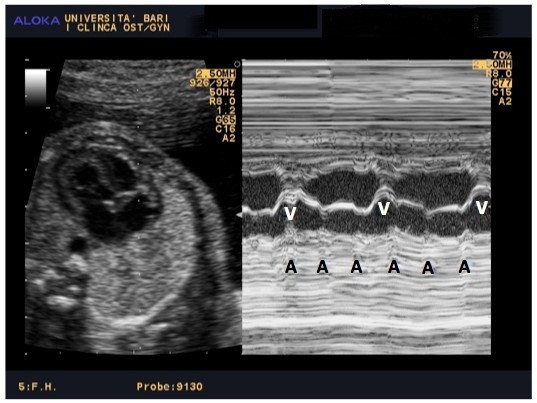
Ultrasonograms of two-dimensional foetal echocardiograpy. Atrial (A) and ventricular (V) contractions.
